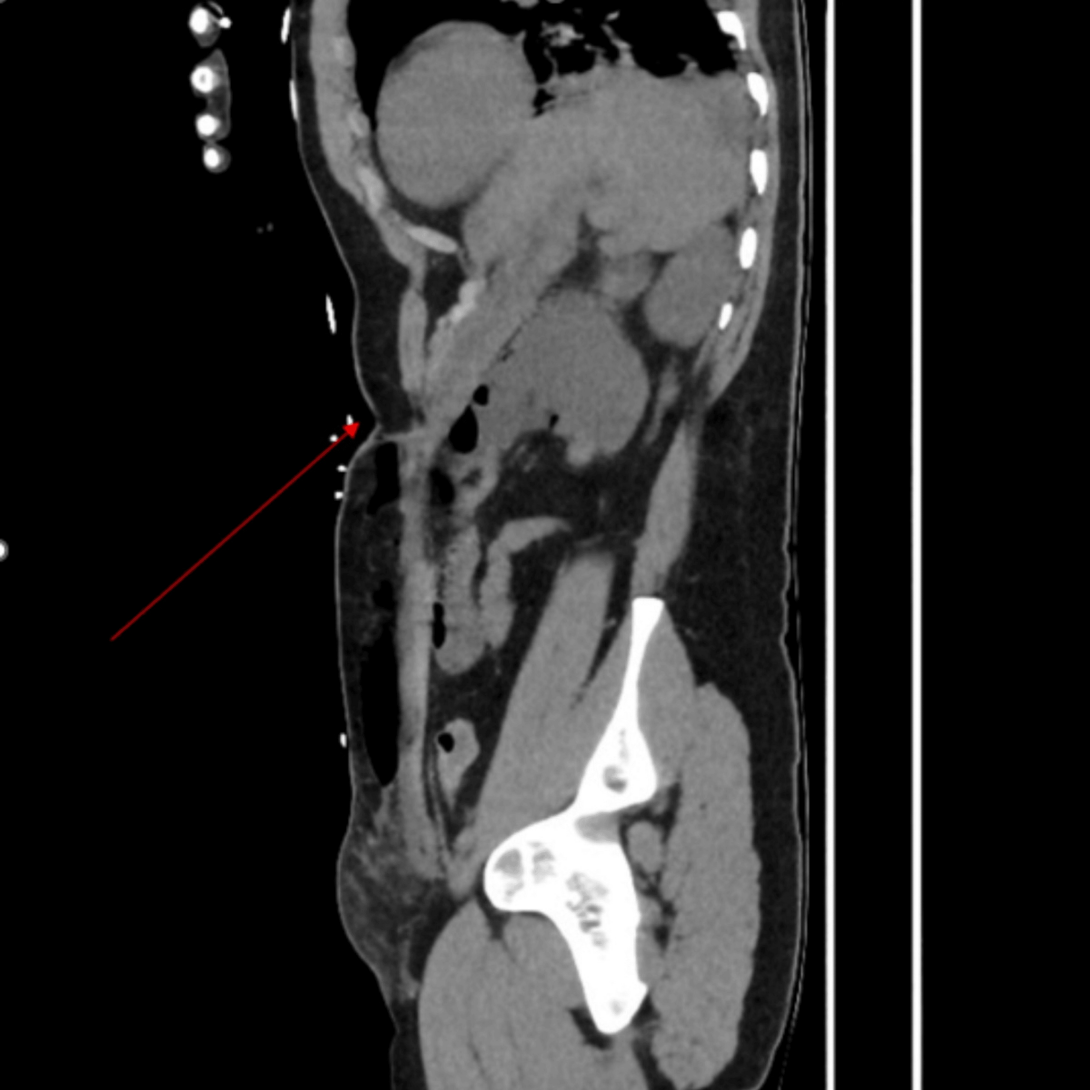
Sagittal view of computed tomography (CT) abdomen and pelvis demonstrating previous percutaneous endoscopic gastrostomy (PEG) insertion site (highlighted by a red arrow) and perforation of the stomach into the subcutaneous space, evident by gas locules within the subcutaneous layer above the anterior abdominal wall.
